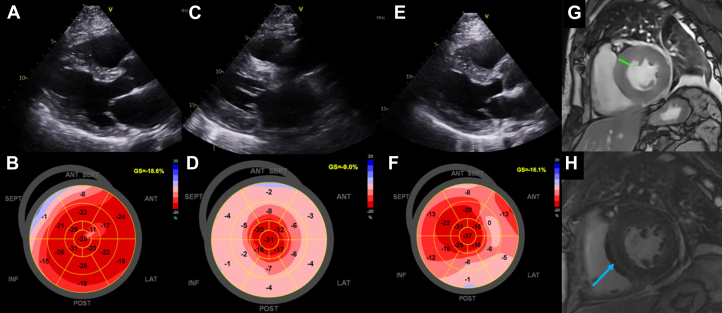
Cardiac Imaging at Baseline and Post-Cyclophosphamide Administration Baseline echocardiogram (TTE) with normal left ventricular (LV) wall thickness (A) and normal global longitudinal strain (B). TTE 8 days after administration of cyclophosphamide with concentric LV wall thickening (C) and reduced global longitudinal strain (D). TTE 18 days after cyclophosphamide administration showing decreased LV wall thickness (E) and improved longitudinal strain (F). Cardiac magnetic resonance imaging on day 8 demonstrated concentric LV myocardial thickening (G; up to 20 mm green line) and nonischemic, mid-myocardial late gadolinium enhancement (H; blue arrow).
